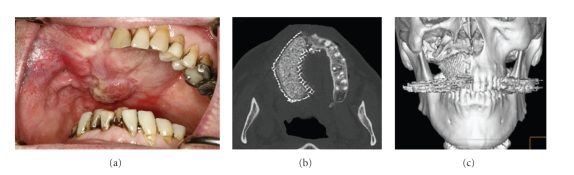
Two months postoperative results of the study by Mesimaki et al. reconstructed a major maxillary defect in an adult patient using autologous adipose-derived MSCs (ASCs) combined with rhBMP-2 and *β*-TCP granules in a microvascular reconstruction surgery. Two months postoperative results indicate that (a) the rectus abdominis muscle has atrophied nearly totally and epithelialized almost completely. Only a small area in the molar region was nonepithelialized. A well-formed buccal sulcus is also noted. Axial (b) and 3D CT scans (c) show the shape and normal bone density of the new maxilla.
